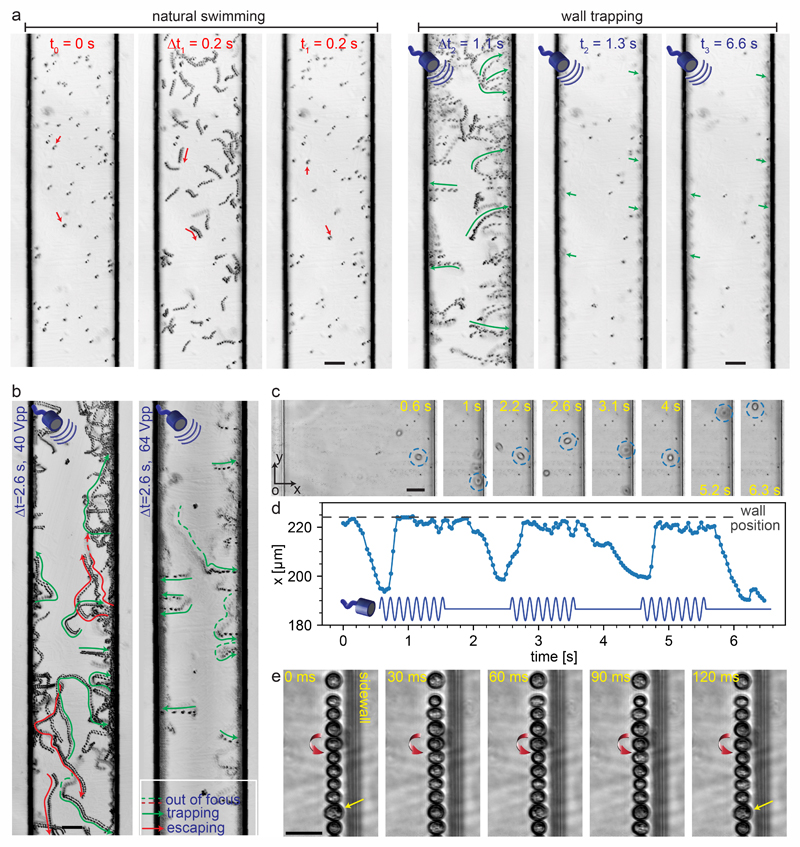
Microalgae-wall interactions under ultrasonic trapping forces. (a) Time-lapse images show trajectory of microalgae during 0.2 seconds natural swimming interval to the wall trapping stage during the next 6.4 seconds (Movie S3†). (b) The effect of the driving voltage amplitude on the trapping of microalgae swimmers, the green and red arrows show the trapping and escaping trajectories, respectively (Movie S4†). (c) The time-lapse images of the single microalgae under pulsed ultrasound signal of 2 seconds width, with its center position in *x*-axis plotted in (d) (Movie S5†). (e) The rotational behavior of the microalgae at the wall boundaries, with a direction denoted by red arrows (Movie S6†). The scale bars in (a) and (b) are 50 μm, and in (c) and (e) are 25 μm.
